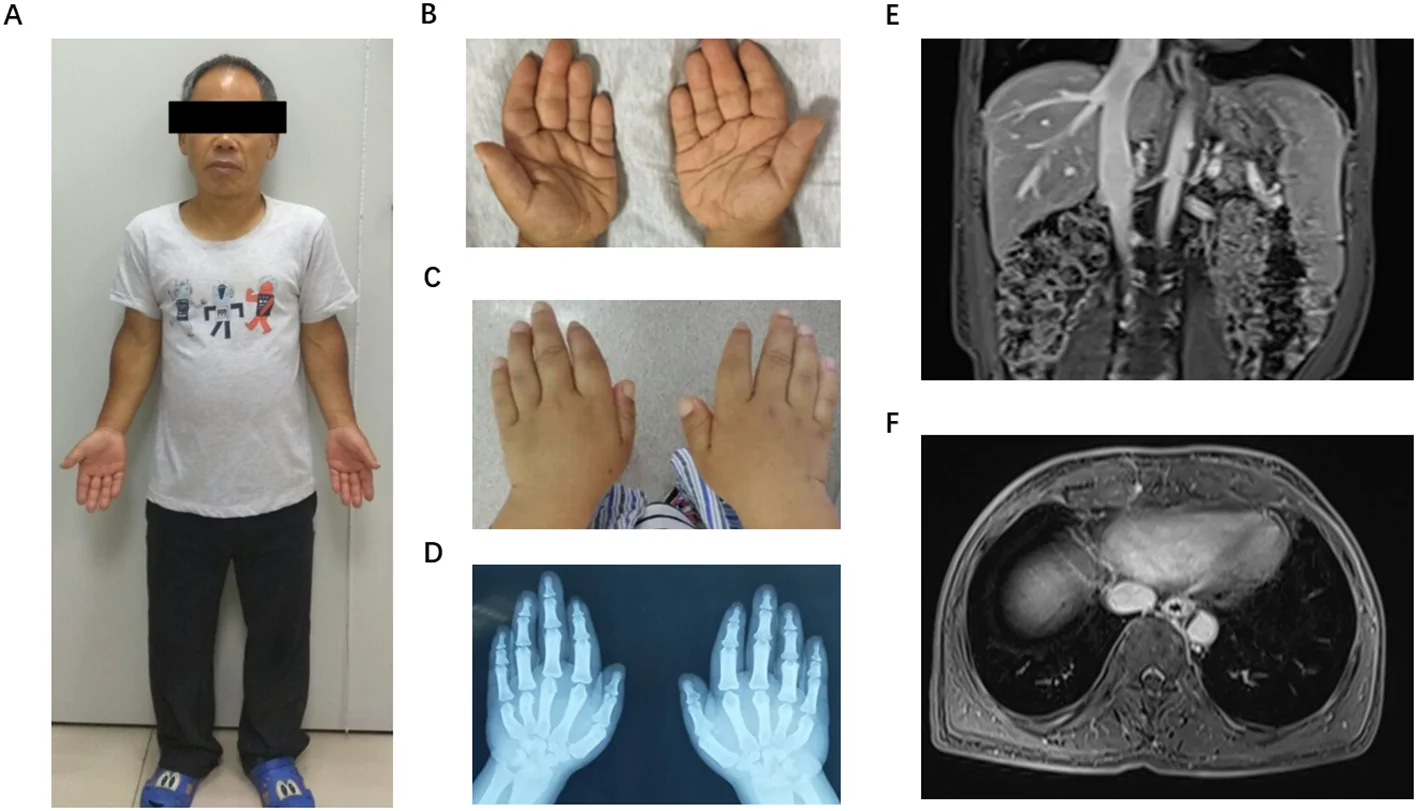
Clinical phenotypes and hepatic magnetic resonance imaging (MRI) findings of the proband. (A) General appearance of the proband, presenting with short stature; (B) Hand photograph of the proband, showing typical brachydactyly; (C) Hand photograph of the proband’s daughter; (D) Hand radiograph of the proband’s daughter, demonstrating marked shortening of the phalanges and metacarpals; (E) Contrast-enhanced hepatic MRI of the proband, showing manifestations of liver cirrhosis including an irregular hepatic surface and heterogeneous parenchymal signal intensity, with concomitant splenomegaly; (F) Contrast-enhanced hepatic MRI of the proband, revealing esophagogastric varices.
